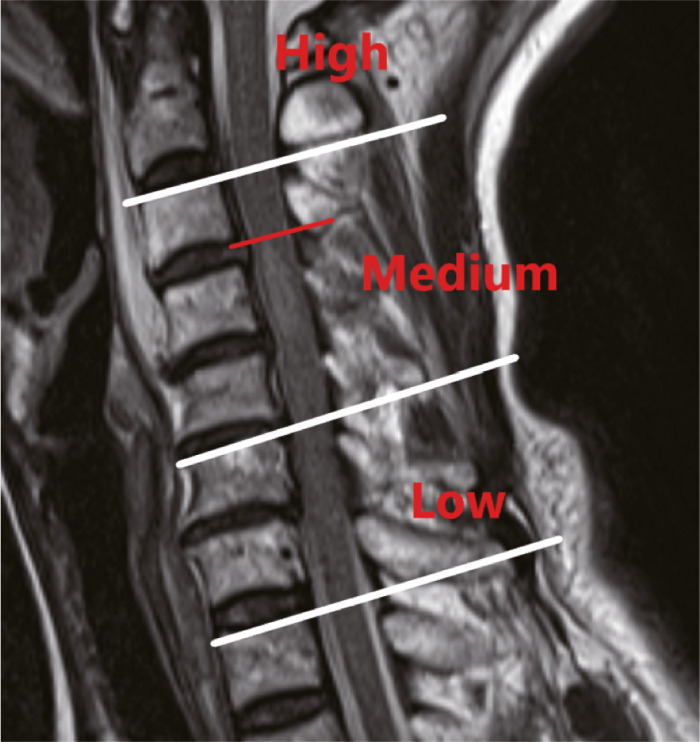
T2‐WI MRI showing edema in the median sagittal plane at the headmost part of the spinal cord above the superior endplate of C3 was defined as a high injury, and edema from the superior endplate of C3 to the superior endplate of C6 was defined as a medium injury, and edema from the superior endplate of C6 to the superior endplate of T1 was defined as a low injury
